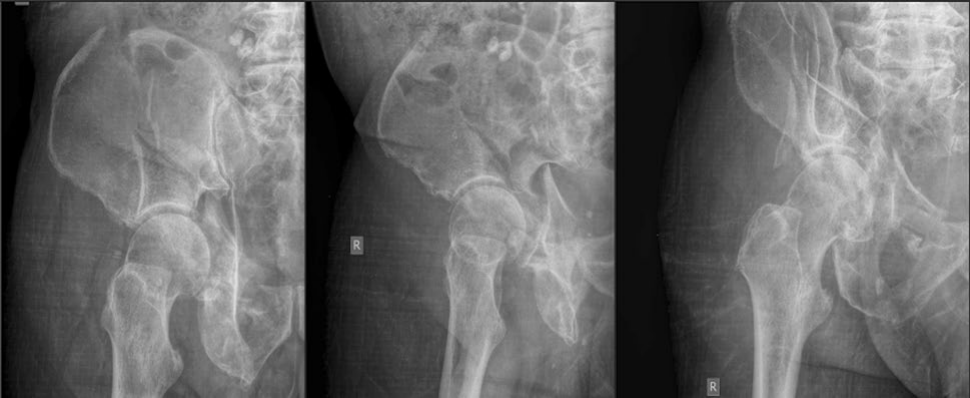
Index radiographs of typically encountered elderly comminuted acetabular fracture: index AP and Judet views
